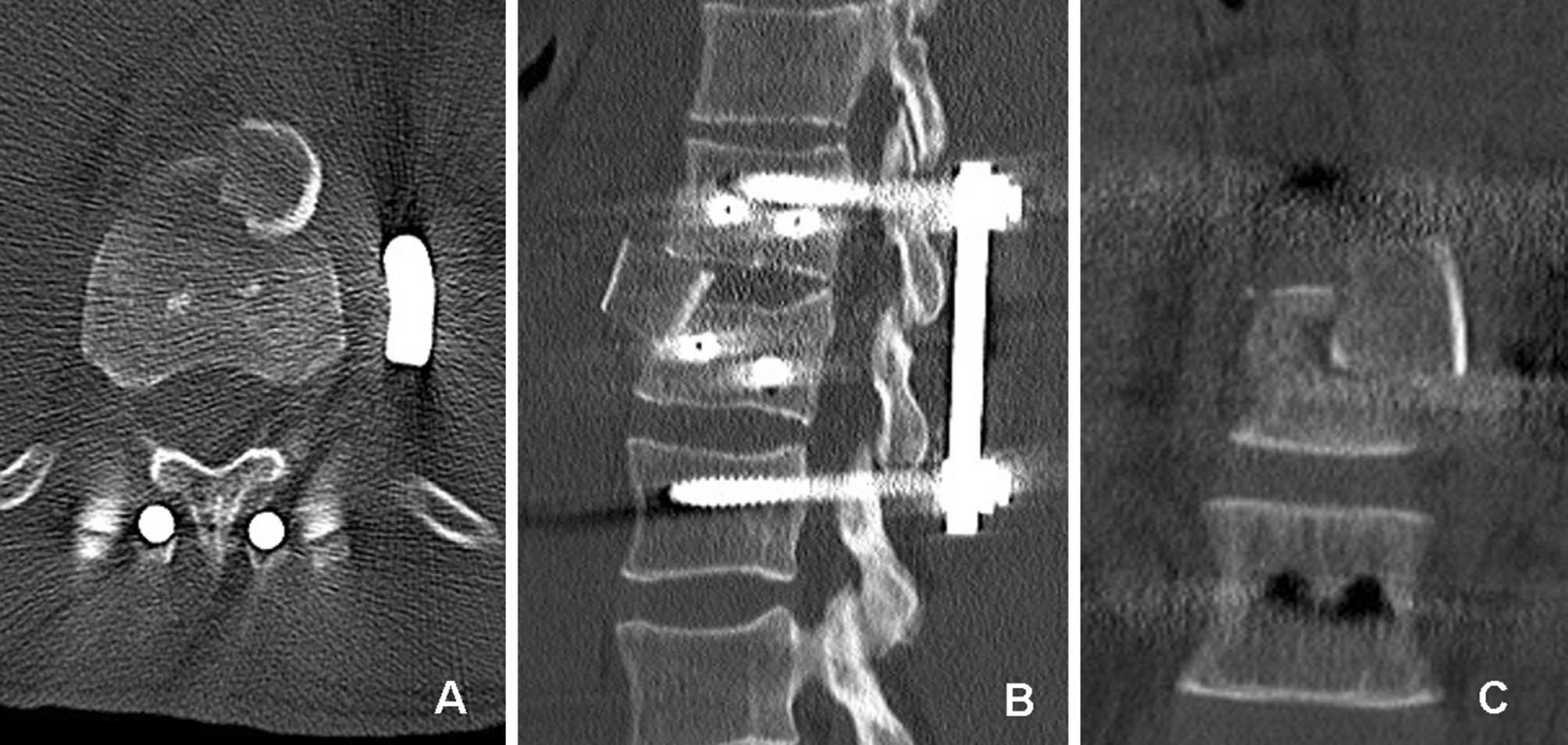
Postoperative CT of the thoracolumbar junction showing a dislocation of the iliac crest bone graft, which was caused by the caudal-anterior screw of the plating system.
